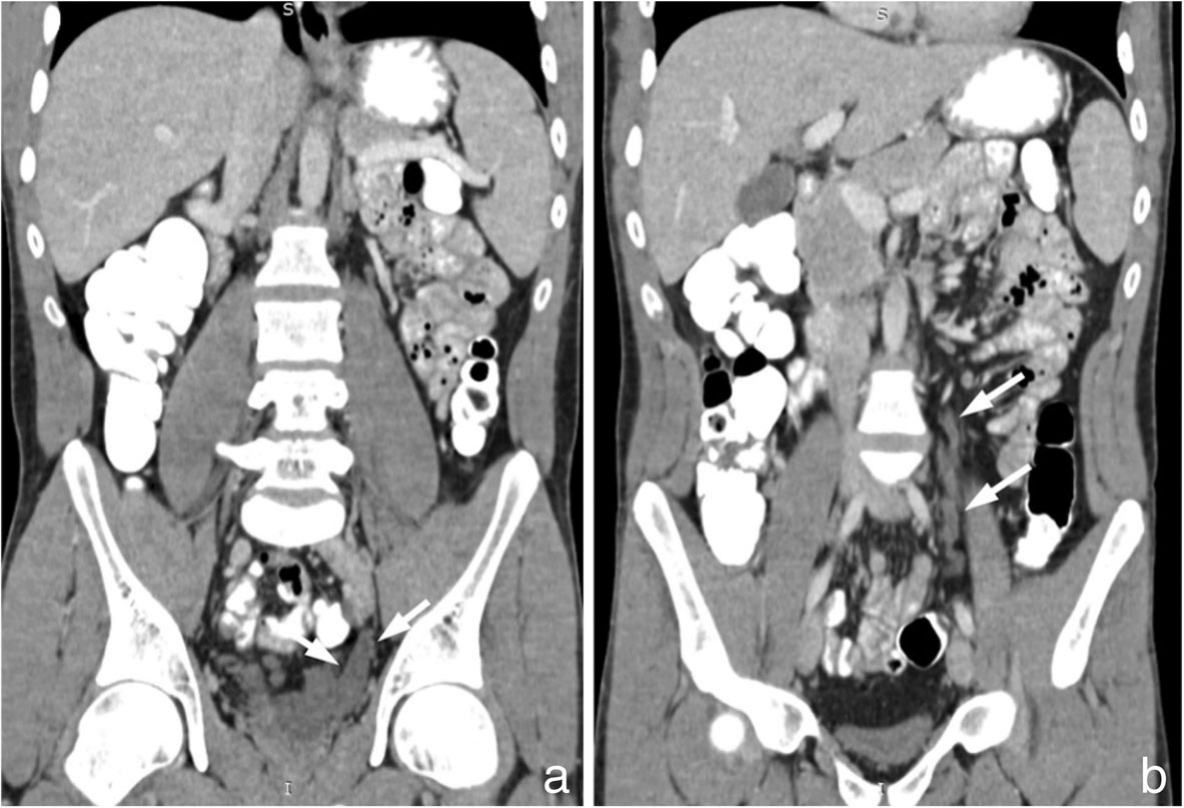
Contrast-enhanced coronal reconstruction CT images of the abdomen and pelvis depict a saccular dilated ectopic ureter opening into the left cystic seminal vesicle (a) and extending centrally up to the level of L_3_ vertebral body (b) (arrows)
